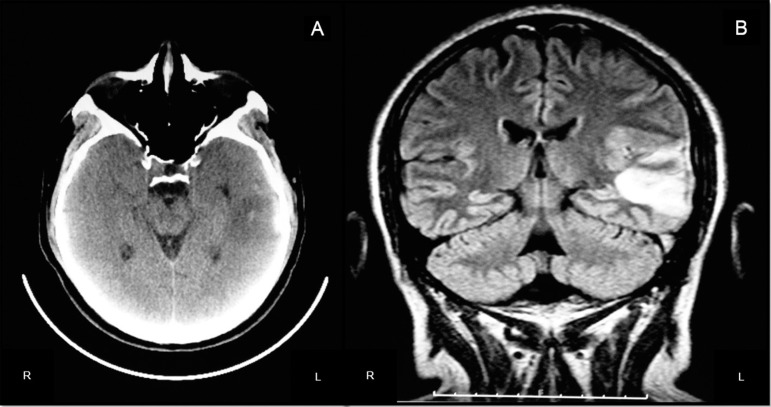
[A] Brain CT from admission showing small hyperdense spots within
